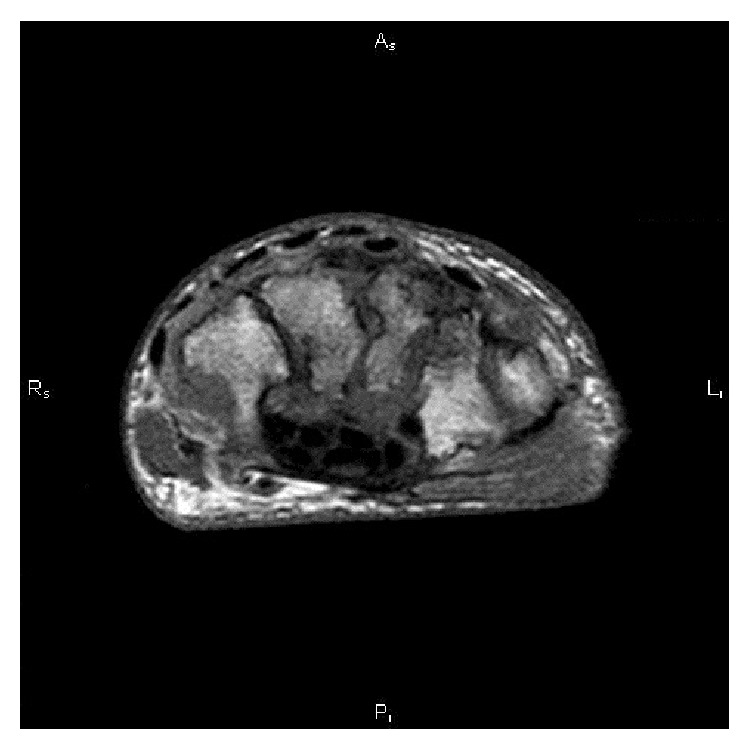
Magnetic resonance imaging of the left wrist before starting antibiotic therapy for* Brucella *spp. and* Mycobacterium bovis*. The picture shows increased signal intensity of trabecular bone of all carpal bones, due to marked medullar edema with many erosive areas, most evident in scaphoid and capitate bones.
